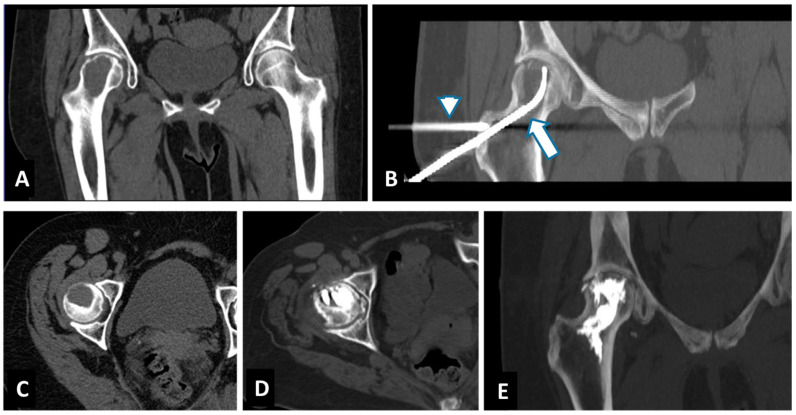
Successful treatment with tRFA and osteoplasty of painful breast cancer metastases of the right femur in a 61-year-old woman. Axial CT and Coronal-CT reconstruction showed a 52 mm lesion located in the head and neck of the right femur (A,C). Coronal-CT reconstruction showed the placements of the steerable bipolar radiofrequency device (tSTAR) (arrow). A second cannula is placed through the greater trochanter to extend osteoplasty (arrowhead) (B). Axial and Coronal-CT reconstruction showed the result after osteoplasty with PMMA (D,E).
